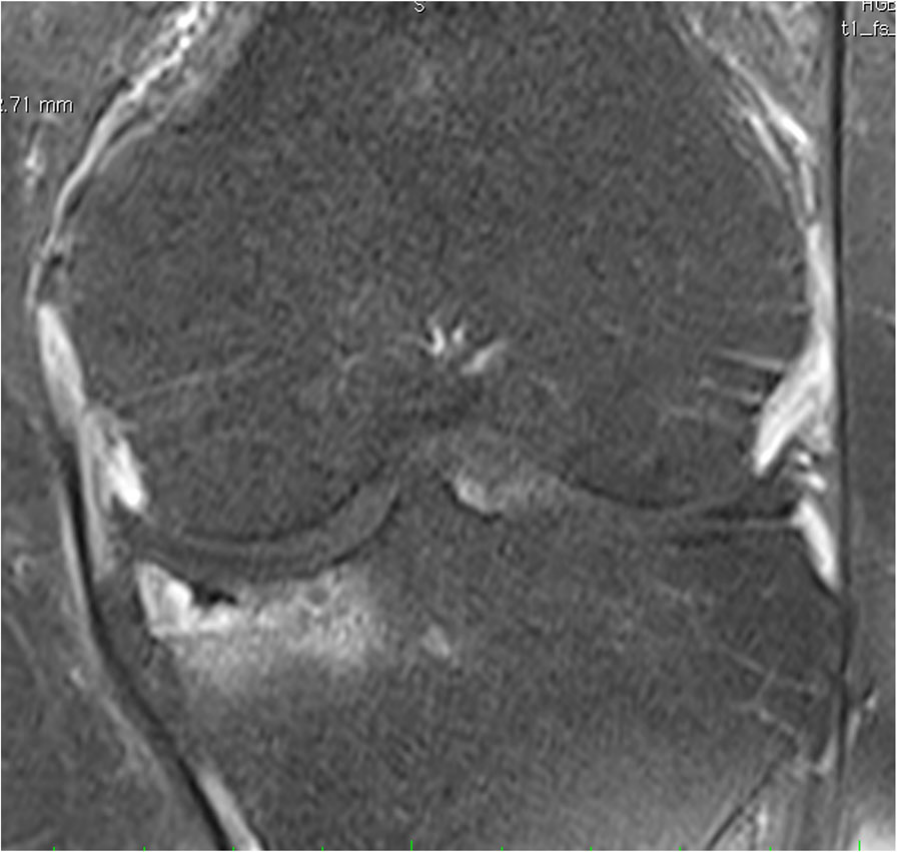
Case 1 patient: postoperative T2-weighted magnetic resonance imaging left knee: progression of the tibial edema, cystic defects and depression of the medial tibia plateau
